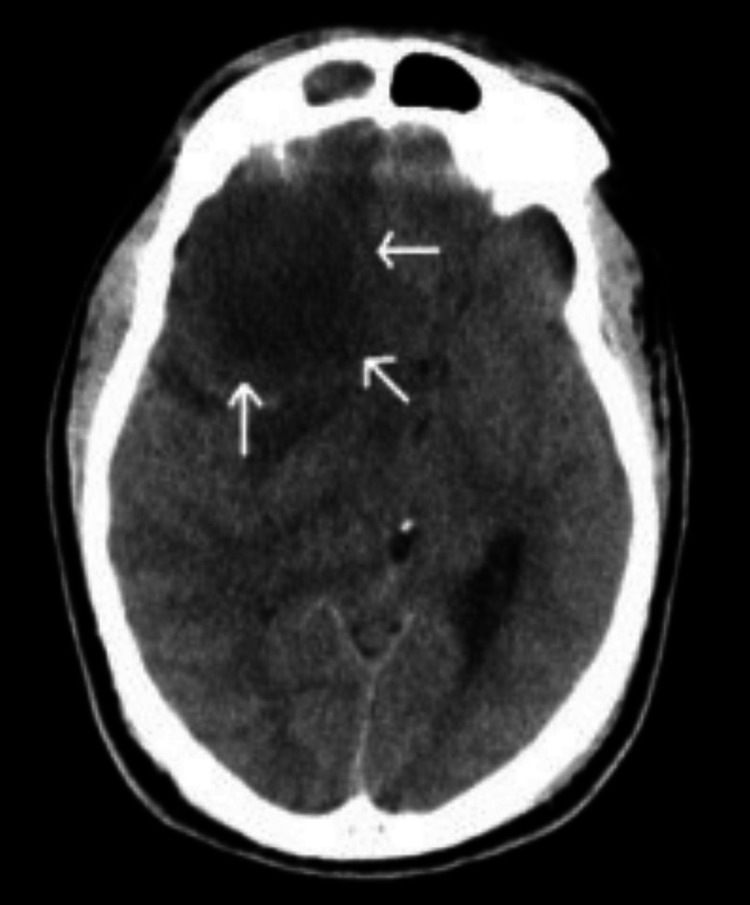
Axial CT (soft-tissue window) demonstrating hypodense right frontal lobe mass with hyperdense rim (arrows).
